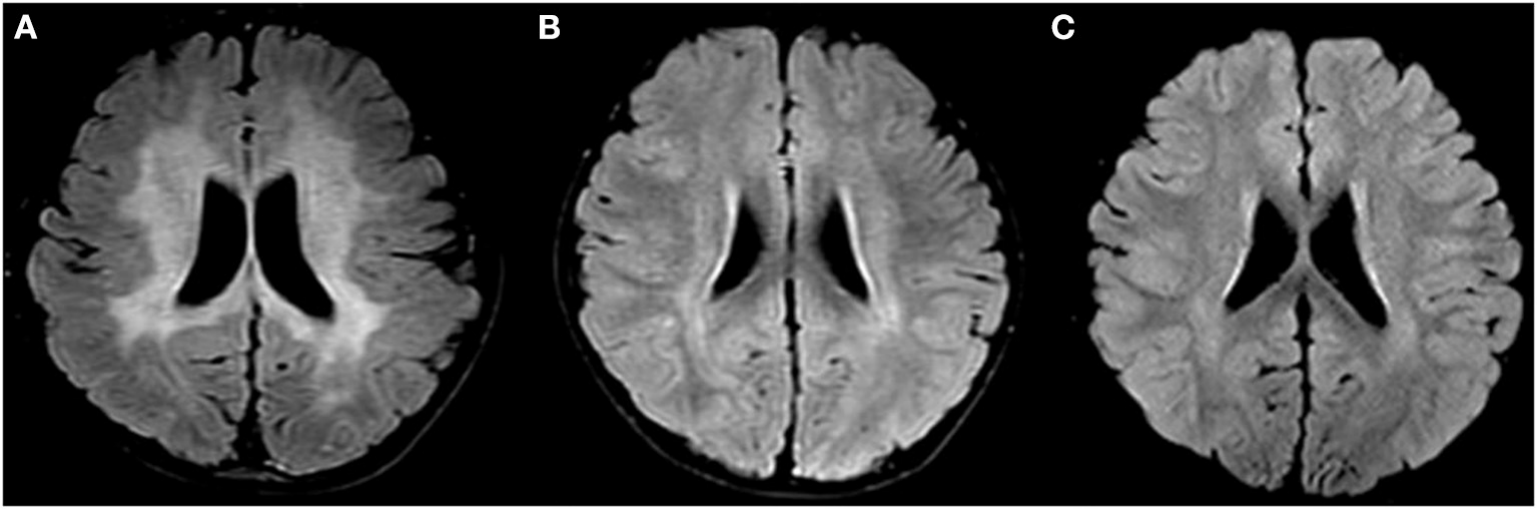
Changes in brain MRIs associated with Zellweger syndrome. (A) Brain MRI of patient 1, A T2-weighted FLAIR image shows high signal intensity (PHILIPS MRI). (B) Brain MRI of patient 2 before transplantation. A T2-weighted TIRM shows high signal intensity (SIEMENS MRI). (C) Brain MRI of patient 2 after transplantation. A T2-TIRM shows high signal intensity (SIEMENS MRI). FLAIR, fluid-attenuated inversion recovery; TIRM, turbo inversion recovery magnitude.
